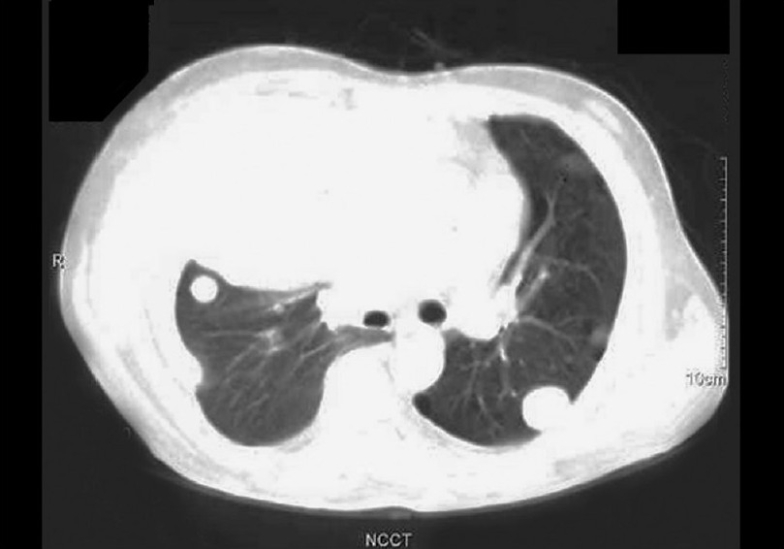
CT scan showing mass lesion in right upper zone with metastasis in both lung fields
